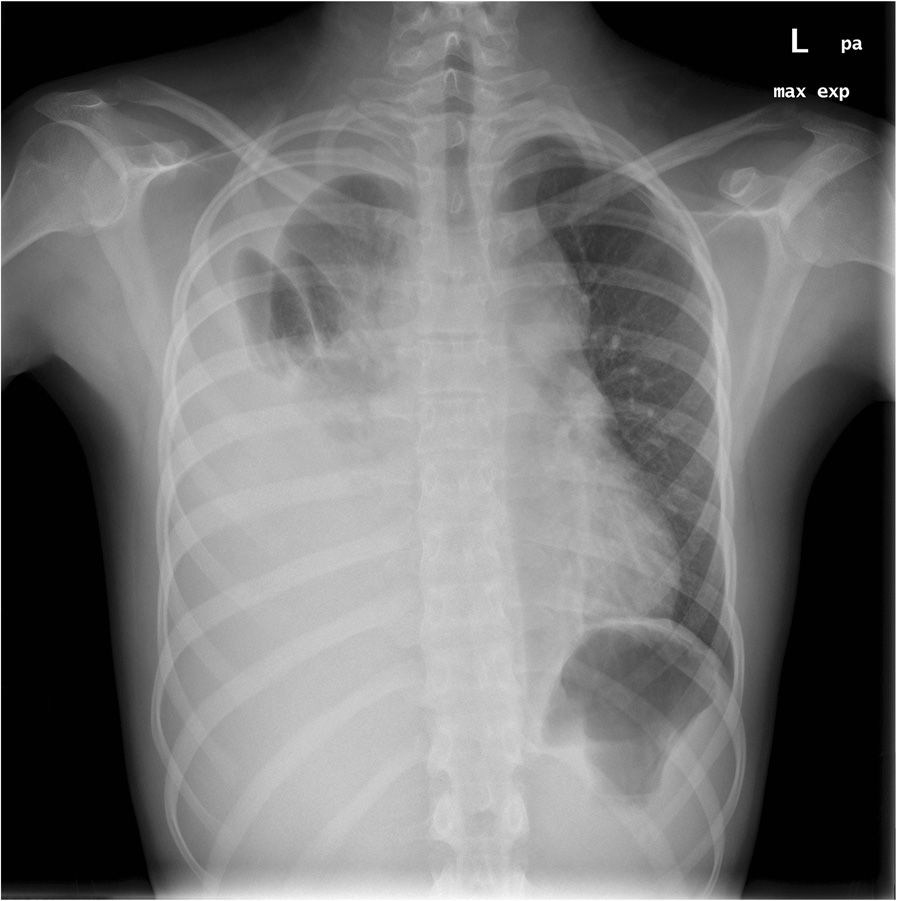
X-ray of thorax. Right side atelectasis, pleural effusion, and broadened mediastinum.
